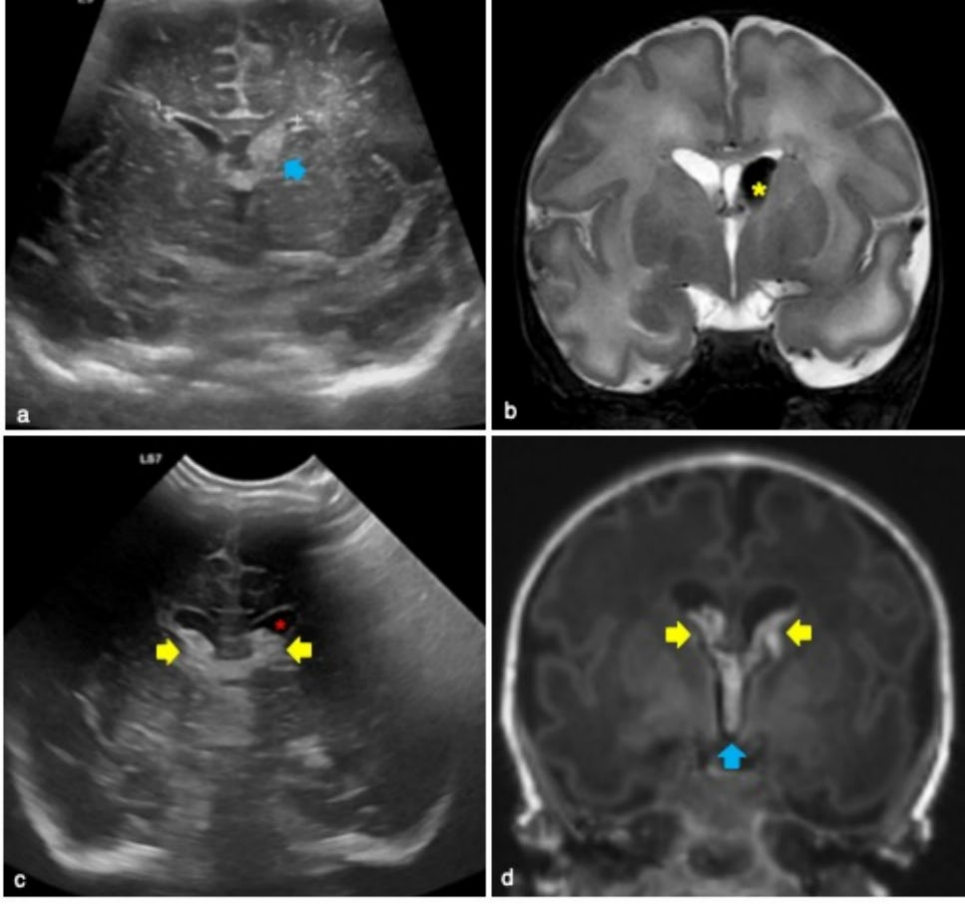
Typical findings of germinal matrix hemorrhage of grade 3. Premature male infant born at 34 weeks’ gestation who underwent cerebral ultrasound at 4 days of life showing left subependymal germinal matrix hemorrhage of grade 3 on coronal plane (blue arrow in a). This finding was confirmed on T2 weighted image by MR (yellow asterisk in b). Premature male infant born at 33 weeks and 5 days gestation who underwent cerebral ultrasound at first day of life showing both lateral ventricular hemorrhages and ventriculomegaly on coronal plane (yellow arrows and red asterisk in c, respectively). Magnetic Resonance performed to complete evaluation, confirmed intraventricular and third ventricle hemorrhages on coronal T1 weighted (yellow and blue arrows in d, respectively)
